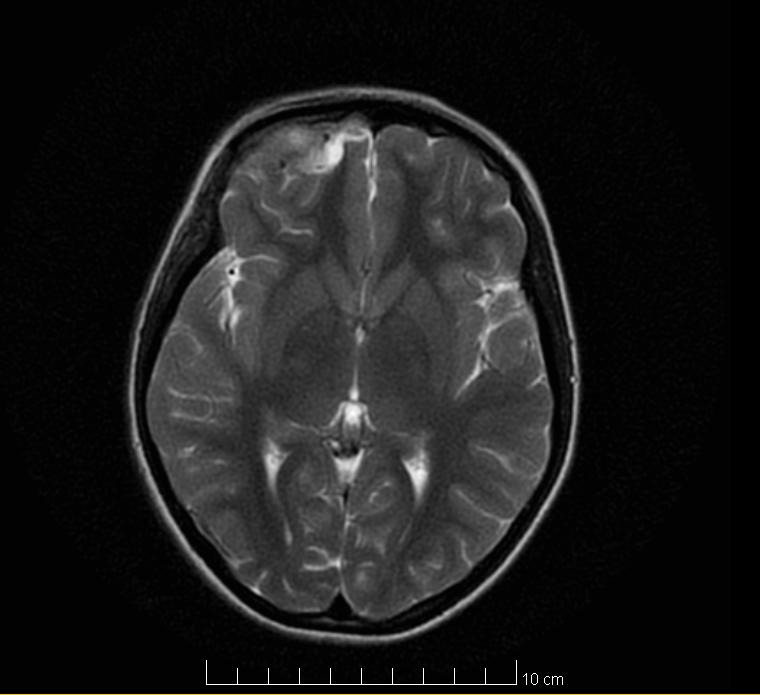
Postoperative Head MRI (yellow arrow - BA remission)
